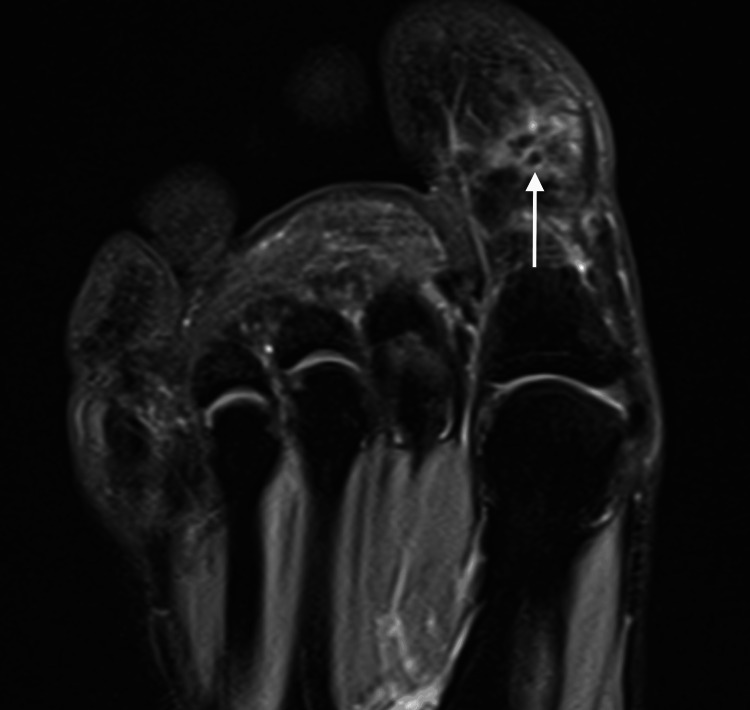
Magnetic resonance imaging. T2-weighted coronal image reveal small subhallucal sesamoid bone (white arrow) with hypointense bone marrow and edema of the adjacent fat planes
